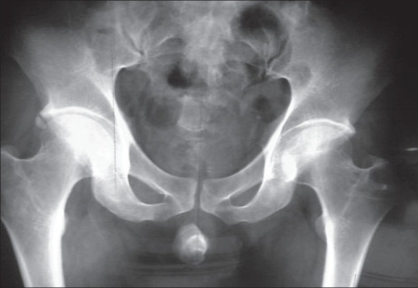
X-ray of the pelvis showing a large urethral stone
